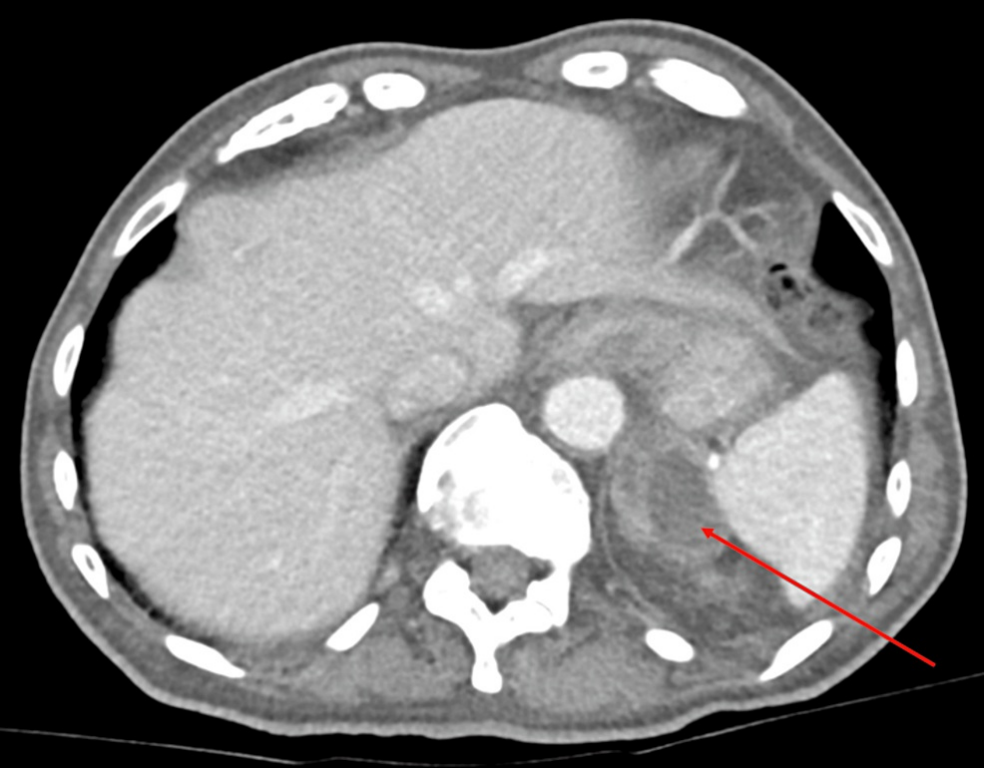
CT abdomen and pelvis with contrast Axial view demonstrating an apparent abscess arising from the left adrenal gland (red arrow); in reality, a gastric diverticulum arising from the posterior fundus of the stomach CT, computed tomography
